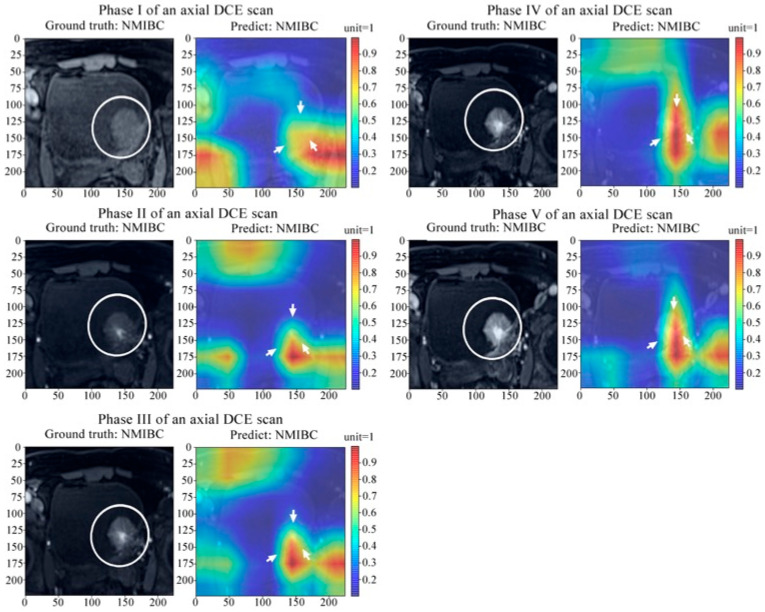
Spatiotemporal attention transition across DCE-MRI phases for non-muscle-invasive bladder cancer characterization, and white elliptical circules and white arrows represent the tumor region in both CT images and the corresponding hot maps, respectively.
